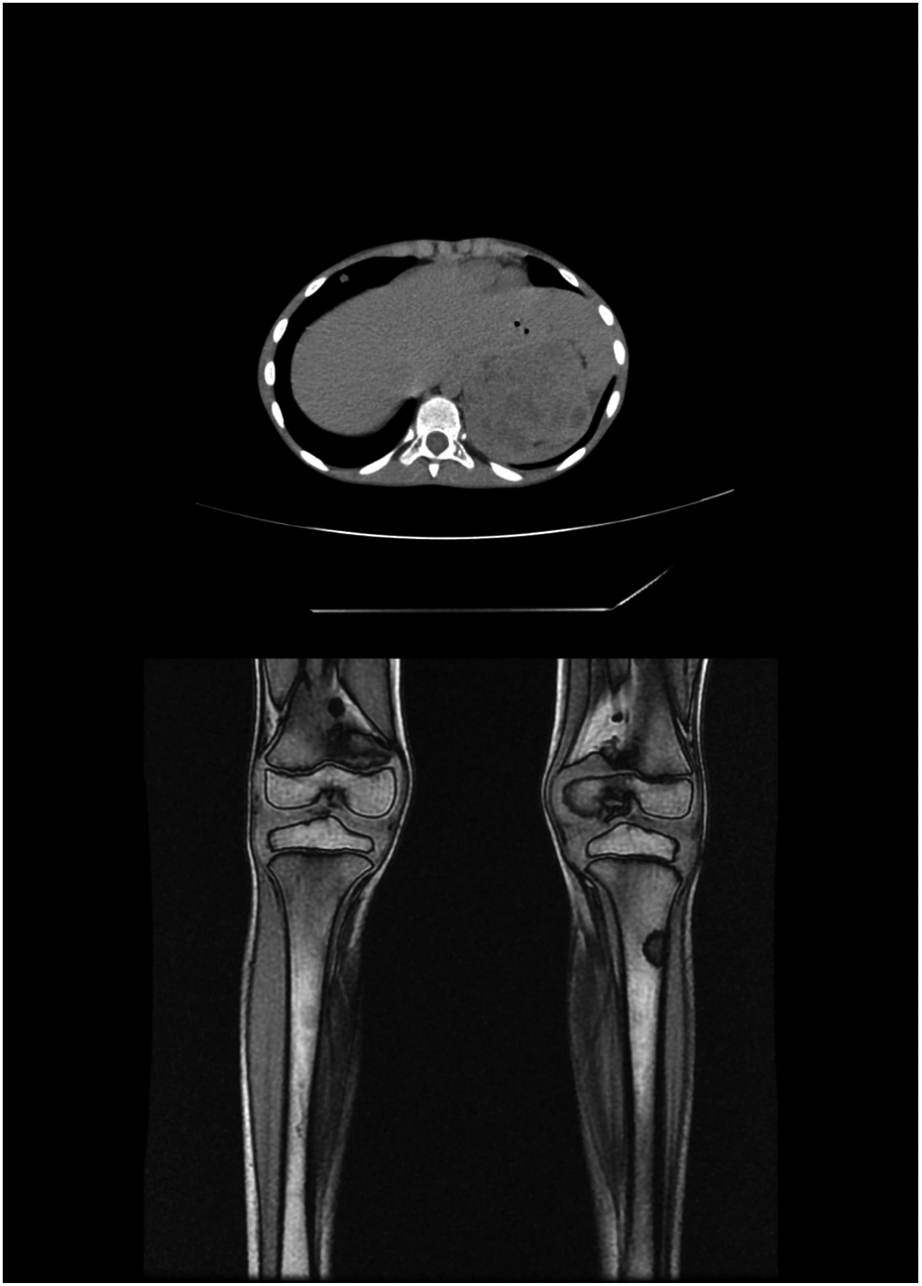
Patient No. 3’s MRI images. MRI, magnetic resonance imaging.
